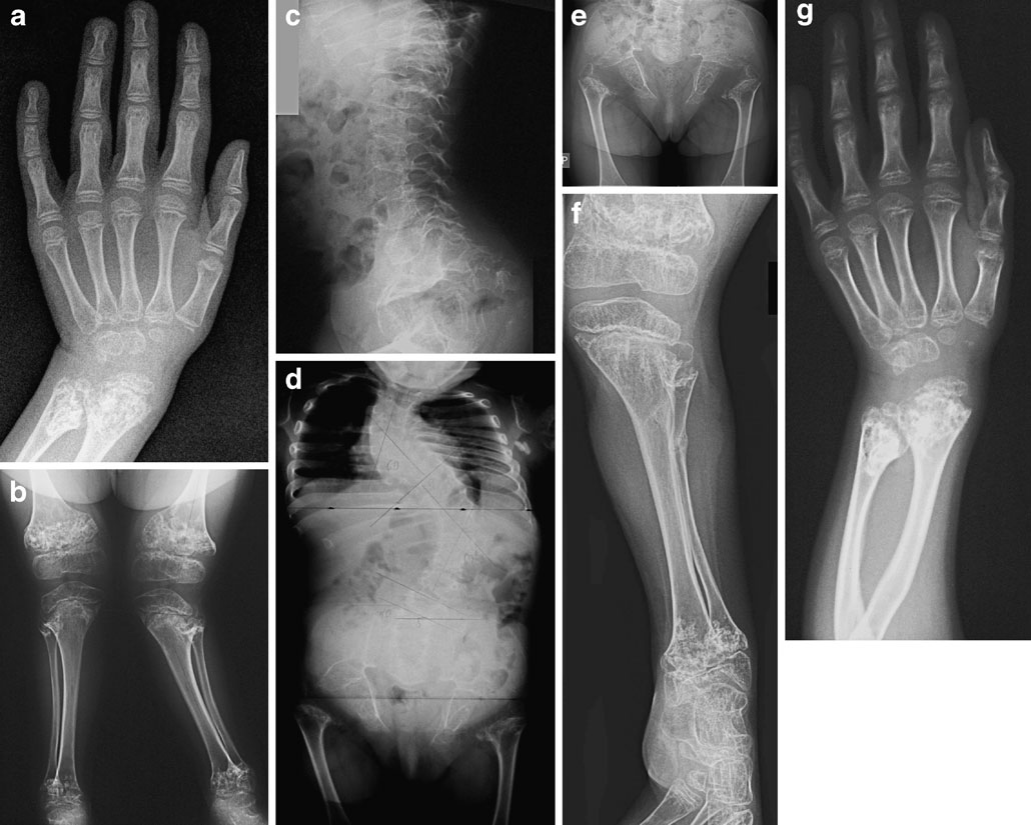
a-g Patient’s radiographs. a. Left hand of the patient at the age 5 years. There is marked metaphyseal involvement of the distal radius and ulna. Dissociation of the carpal bone age with absent ossification of the proximal carpal raw is demonstrated. Only four small carpal ossification centres are present. b. Knees of 8-year-old patient reveal marked metaphyseal involvement and severe coxa valga. c-f Patient at age 10 years. c. Lateral spine with lumbar lordosis and trapezoid shape of vertebral bodies. d. a-p view of spine that shows progress of spinal scoliosis in spite of 6 years of bracing. e. Pelvis. Note osteoporosis, absent capital femoral ossification and delayed ossification of the pubic bones. f. Left leg. Osteoporosis, severe metaphyseal changes and genu valgum deformity are visible. g. Left hand of 11-year old patient. The tubular bones of the hands are slightly affected. There is severe metaphyseal involvement of the forearm bones and dissociation of the delayed carpal bone age with absent ossification of the proximal carpal raw
